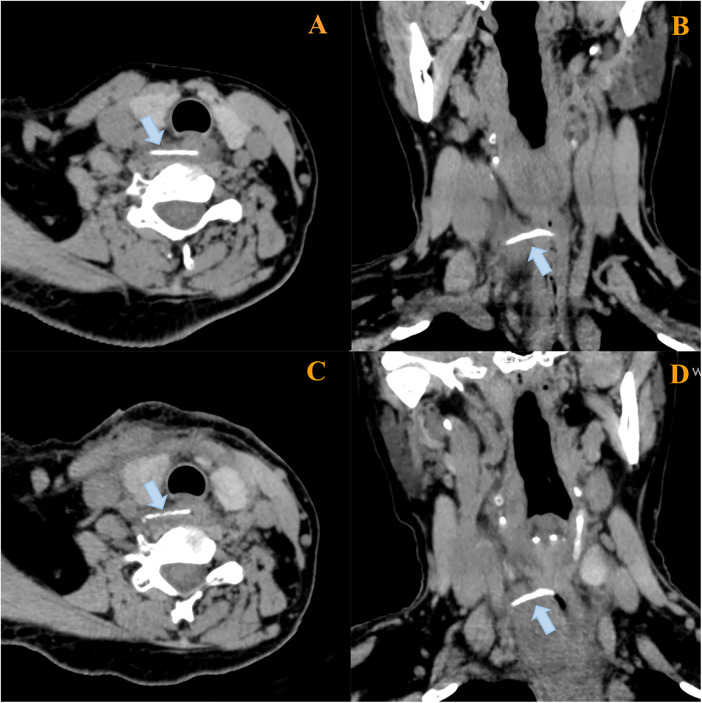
Preoperative and follow-up CT images were acquired without intravenous contrast. Axial and coronal multiplanar reformation (MPR) CT images obtained preoperatively (A,B) and at follow-up (C,D). The preoperative images demonstrate a linear hyperdense foreign body penetrating the wall of the cervical esophageal inlet (at the C6 superior margin) into the right periesophageal space, surrounded by ill-defined fat stranding and patchy exudative densities. The distal end of the foreign body was approximately 3.5 mm from the right common carotid artery. The follow-up images confirm the foreign body’s persistent location. Compared to the initial study, there is increased periesophageal fat stranding and patchy exudation, with the distance to the right common carotid artery measuring approximately 3.2 mm.
